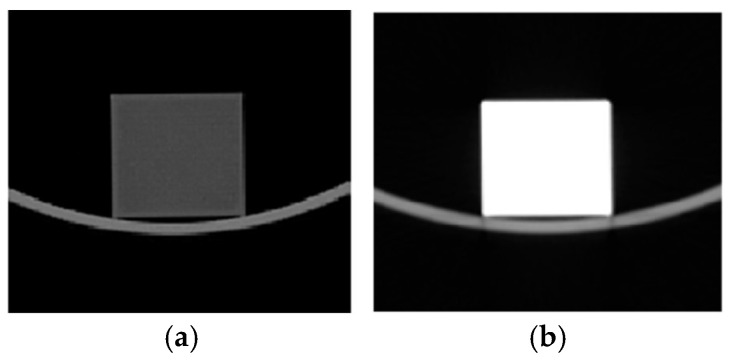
CT scans of experimental samples: (a) ABS, (b) ABS–30Bi_2_O_3_.
